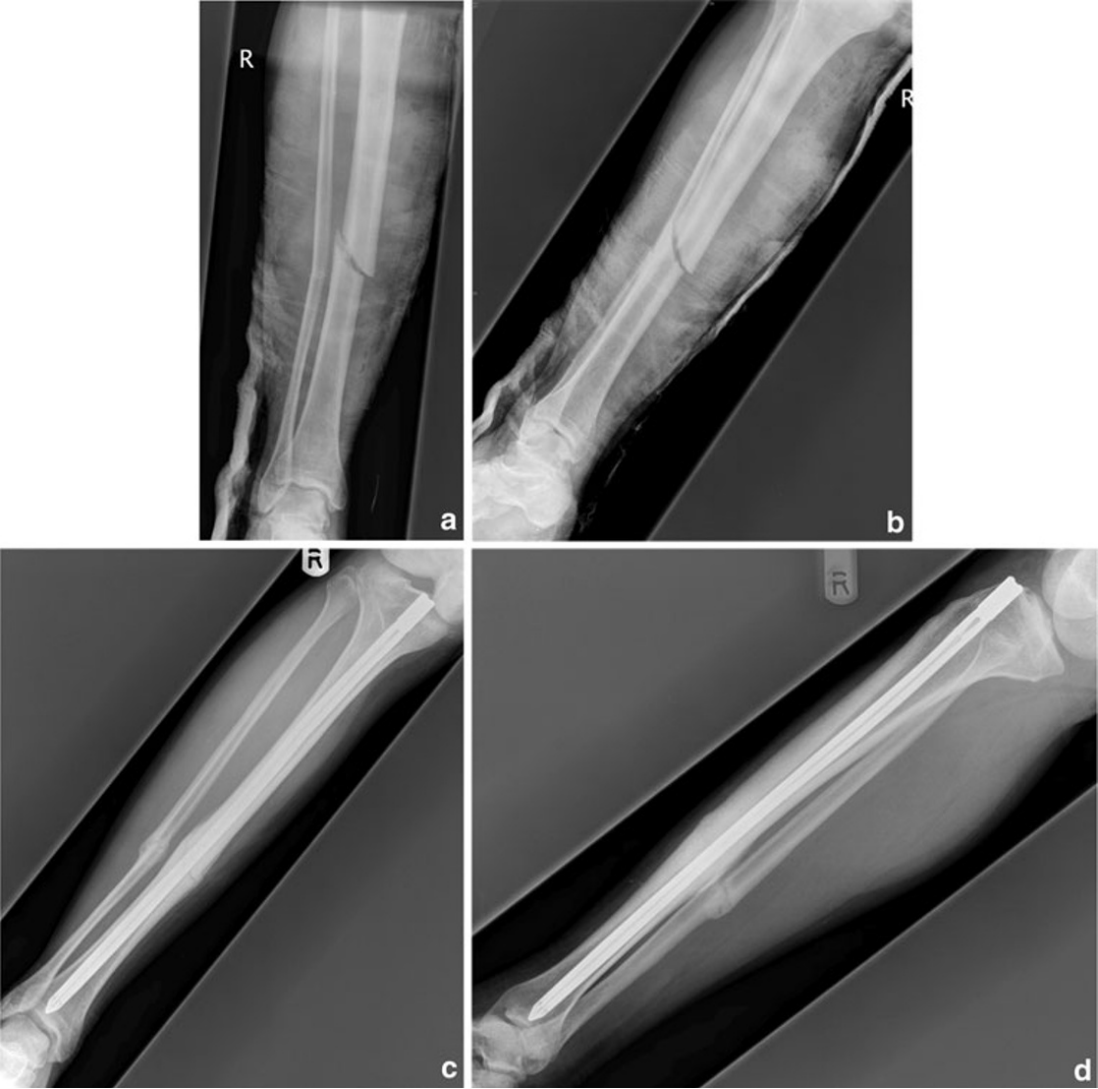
a–d Radiographs demonstrating treatment of an acute tibial shaft fracture with the Fixion™ expandable nail
